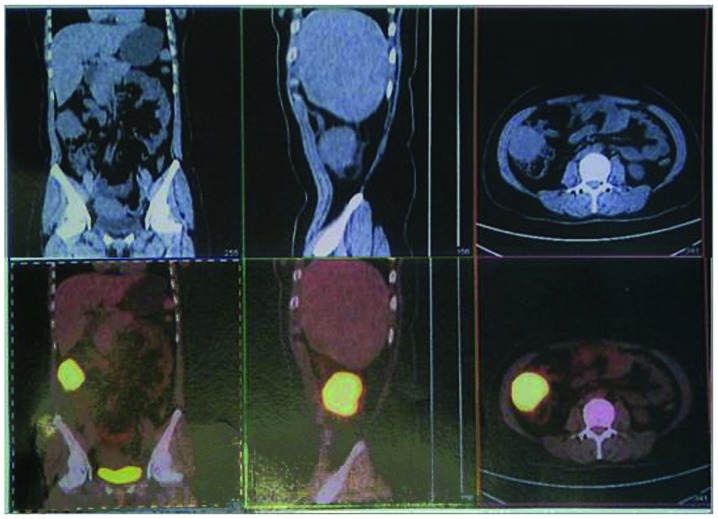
Positron emission tomography/computed tomography scan of the abdomen revealed a large solid mass with hypermetabolism of fluorodeoxyglucose. The mass is highlighted in yellow and was close to the liver.
